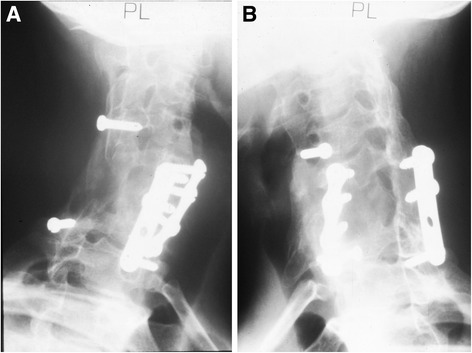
a-b Oblique radiographs of the cervical spine showing a Roy Camille plate positioned to obtain posterior fusion on the left intact side, and 2 screws on the lateral articular masses of C4 and T1 to maintain the graft. An anterior plate stabilized the anterior aspect of the cervical spine
